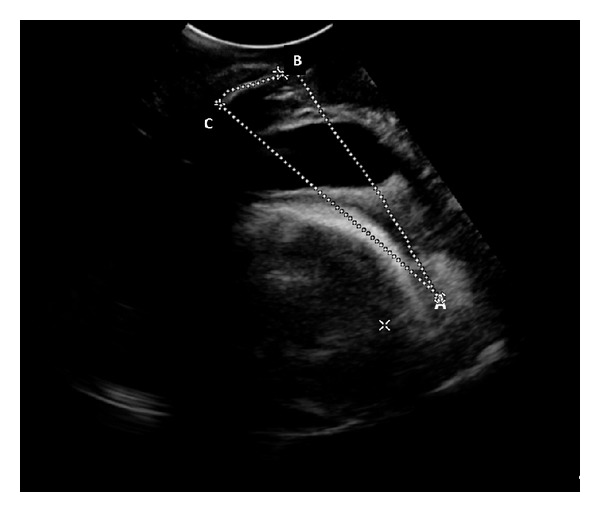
Example of manual contouring performed by an experienced sonographer (standard reference). “A” represents the fetal head outermost point; “B” and “C” are, respectively, the distal and proximal ends of the pubic symphysis.
